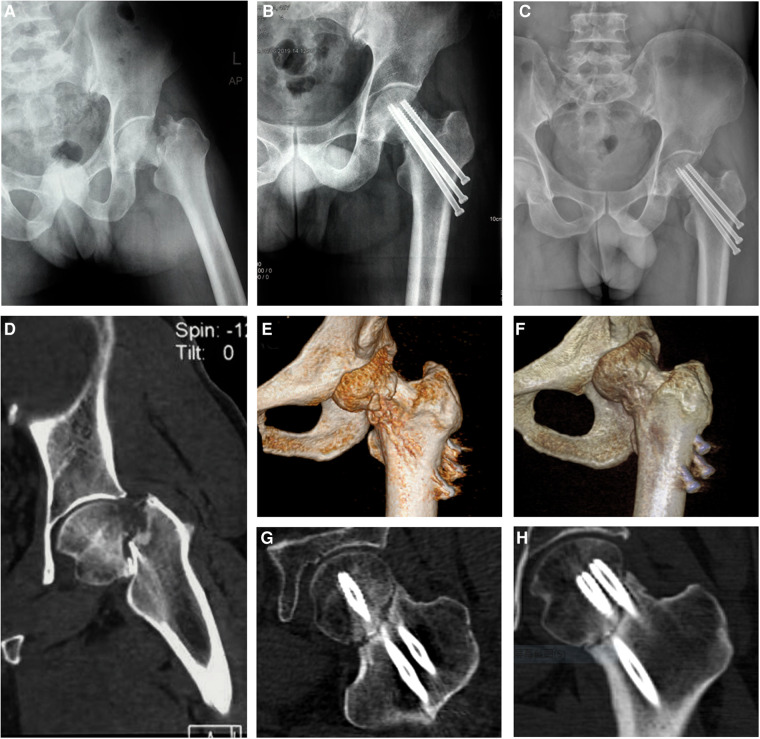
X--ray (A) and CT (D) showing a Garden IV fracture of the left femoral neck. The x-ray (B) and CT (E,G) showing bone resorption at the fracture site with internal fixation loosening and slipping 8 weeks after operation. The x-ray (C) and CT (F,H) showing the non-union of fracture 12 weeks after operation when the patient first visited our hospital and was started on a subcutaneous injection of teriparatide.
